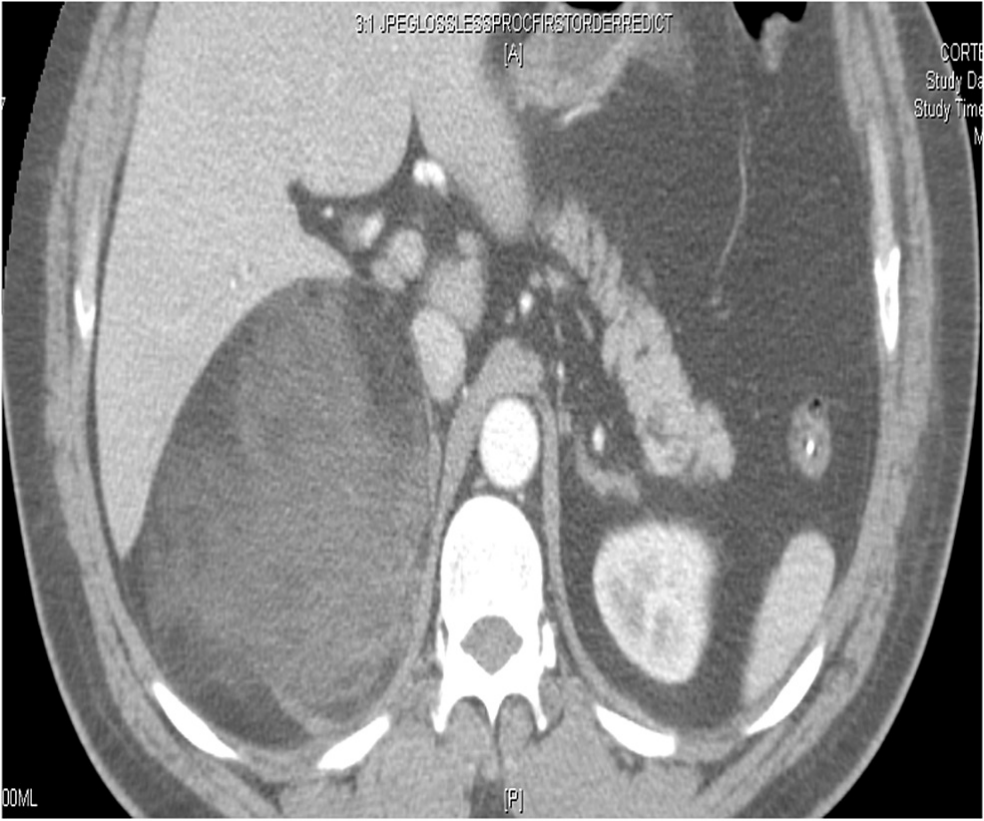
CT Arterial Phase - Axial View Image demonstrates a 15 cm retroperitoneal mass exerting mass effect on the kidney and liver.
